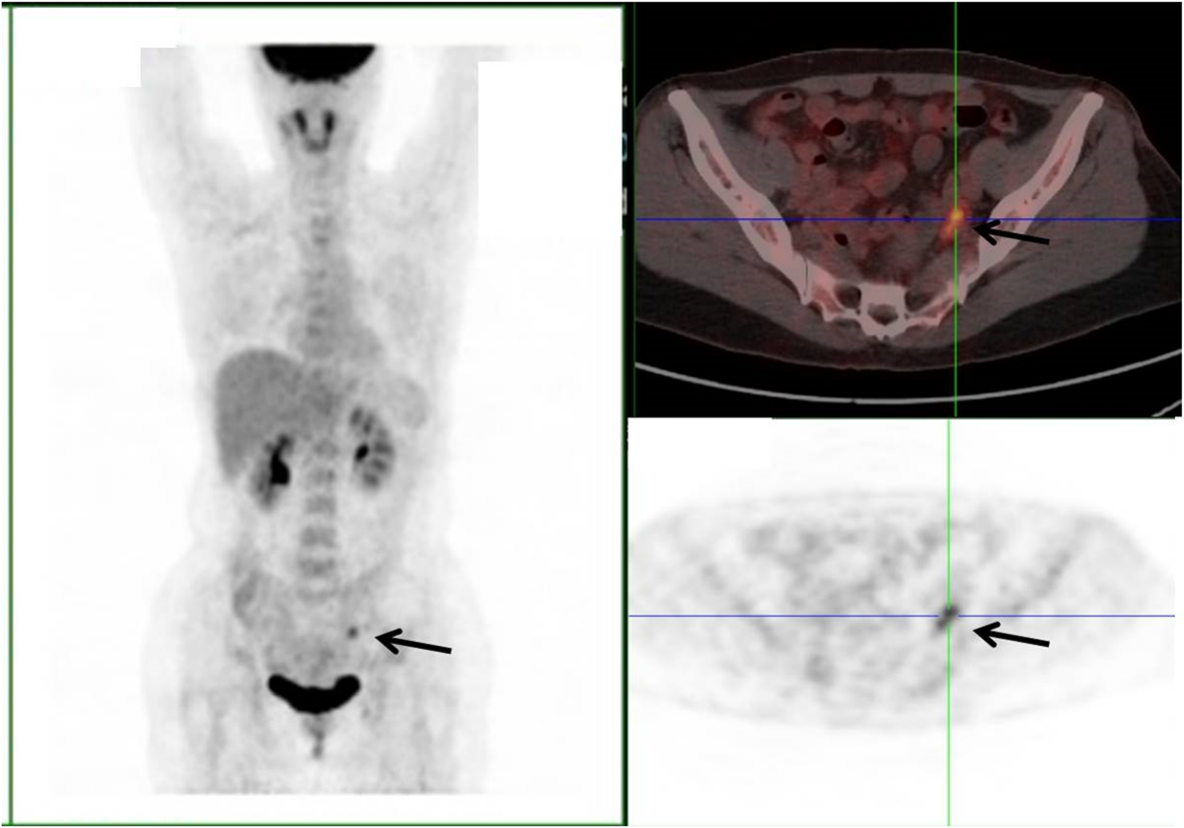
Images of a CUP patient with left iliac vessels and left inguinal lymph node metastasis. The patient had no symptoms such as diarrhea, constipation, or hematochezia. 18F-FDG PET-CT images after left inguinal lymph node surgery showed metastasis in a lymph node lining the iliac vessels identified (black arrow), and he was otherwise normal. Repeat anorectal examination showed a suspicious lesion in the anal canal, which was finally pathologically confirmed by enteroscopy, although the prior enteroscopy showed no abnormal findings
